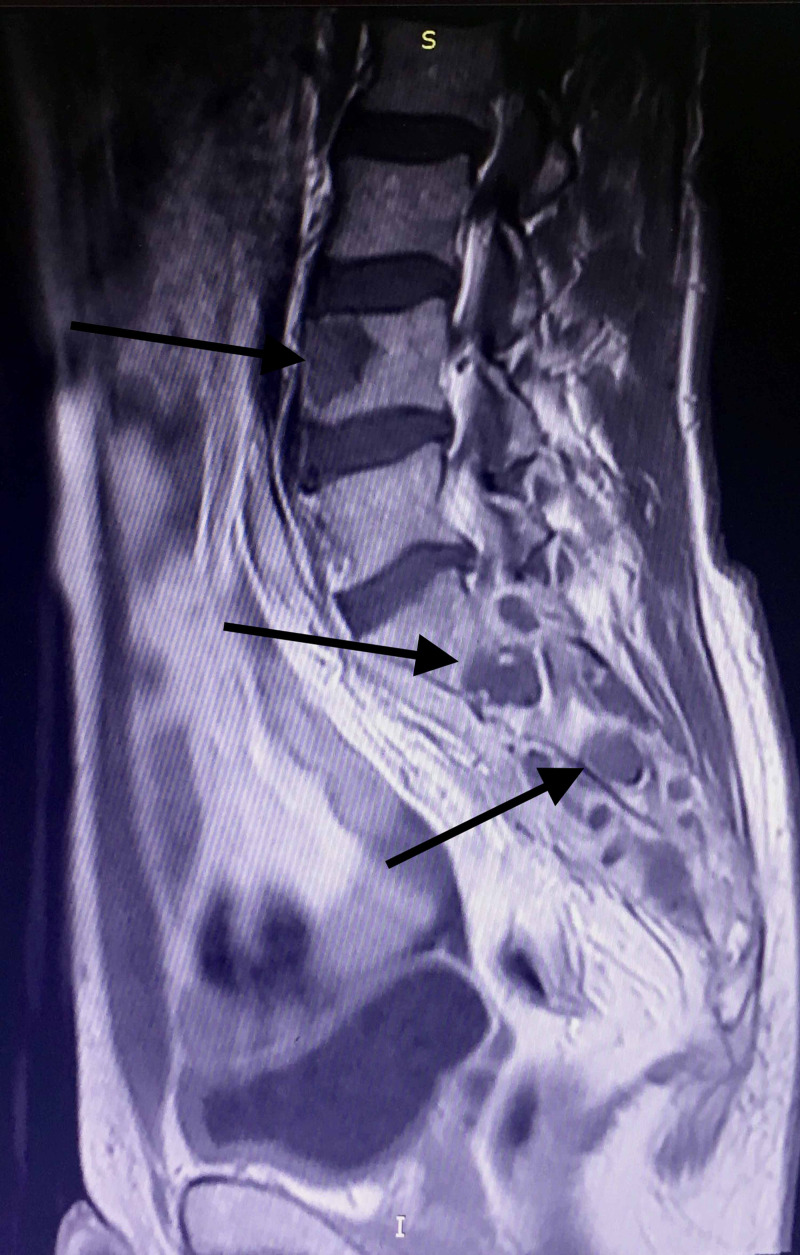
MRI findings, including cystic lesions in the right sacroiliac joint and the L4 vertebra.
